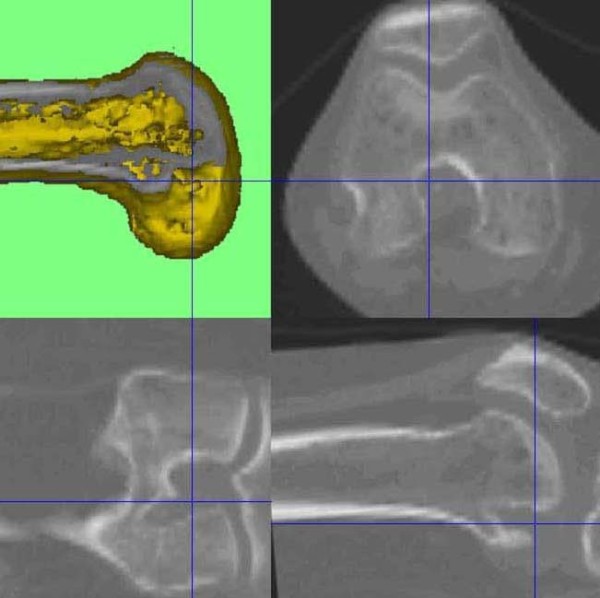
CT out of the SPECT/CT data showing femoral and tibial bone tunnels 3D-CT analysis, which indicate a shallow and high placed ACL graft on the left knee.
